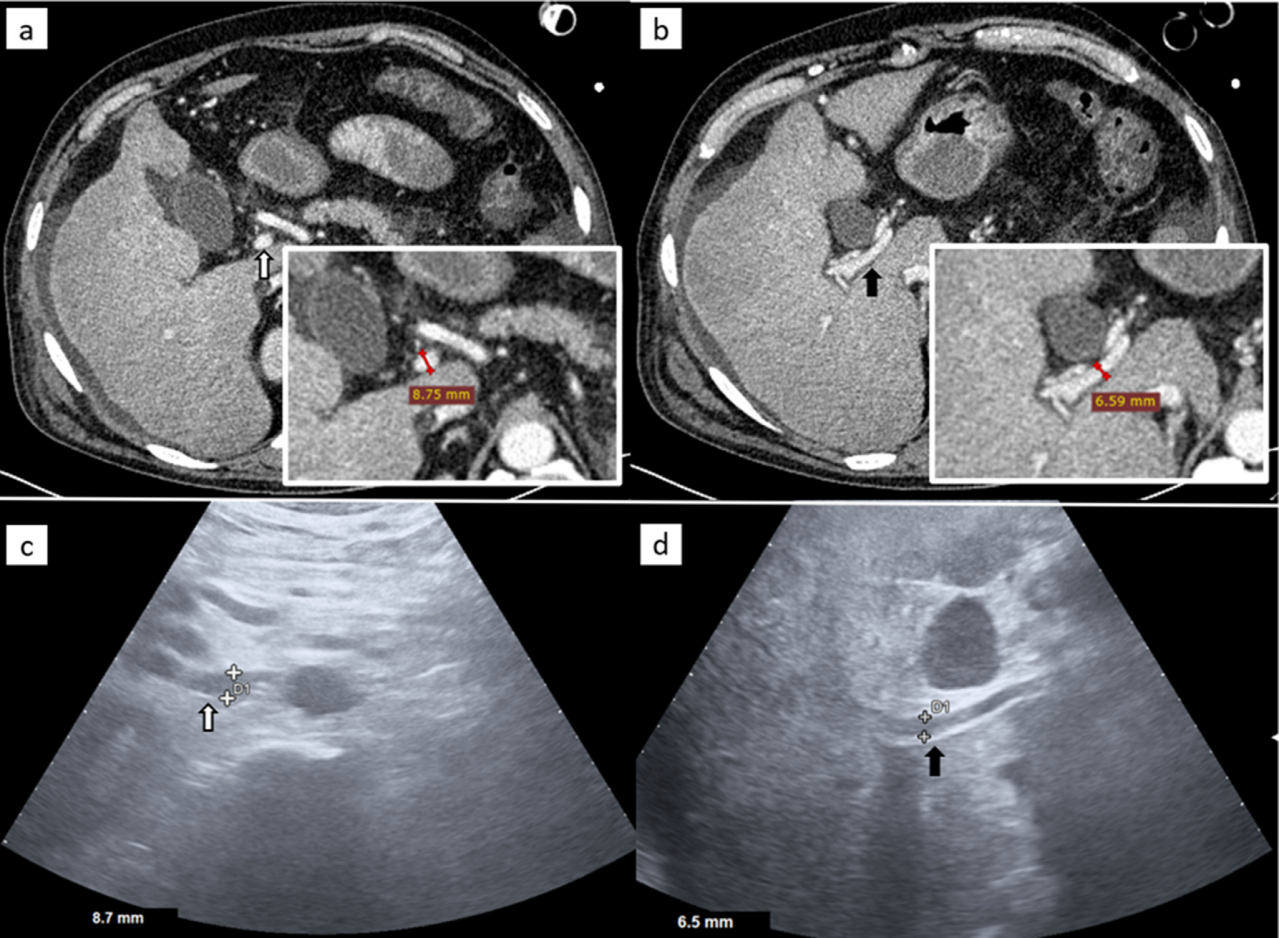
Contrast enhanced computed tomography (CECT) and corresponding ultrasound (US) imaging. White arrow: extrahepatic tract of the portal vein and its measurement in CECT (a) and in US (c). Black arrow: intrahepatic tract of s portal vein and its measurement in CECT (b) and in US (c). Red line: CT caliper; White + and D1: US caliper.
